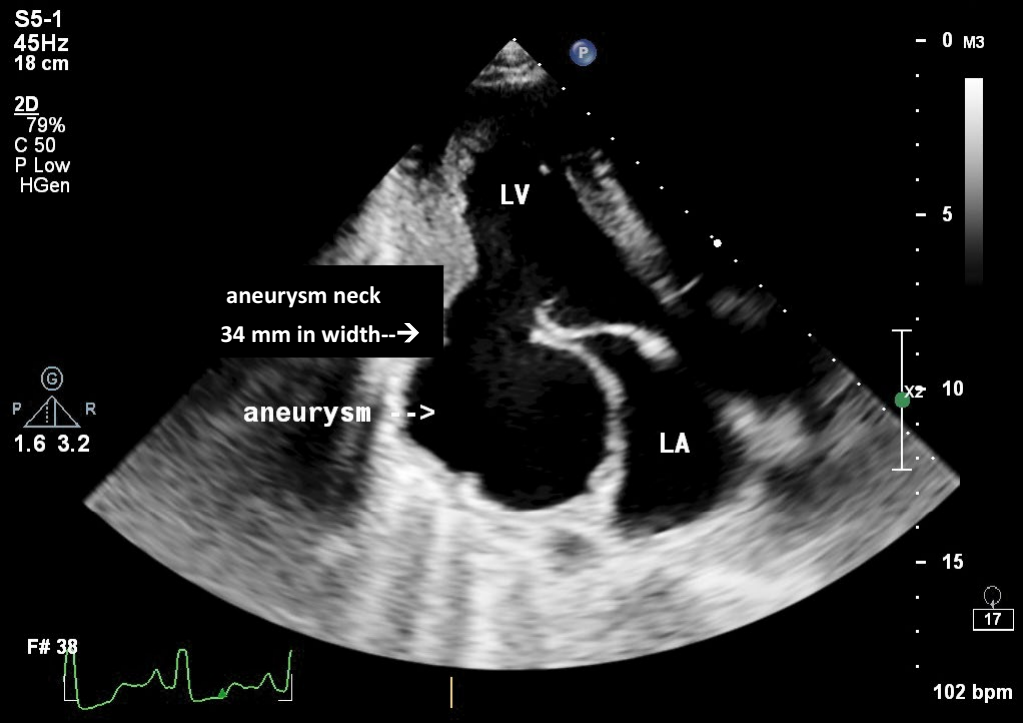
Transthoracic echocardiogram showing the large submitral valve aneurysm and aneurysm neck which is 34 mm in diameter. LA, left atrium; LV, left ventricle; RV, right ventricle
